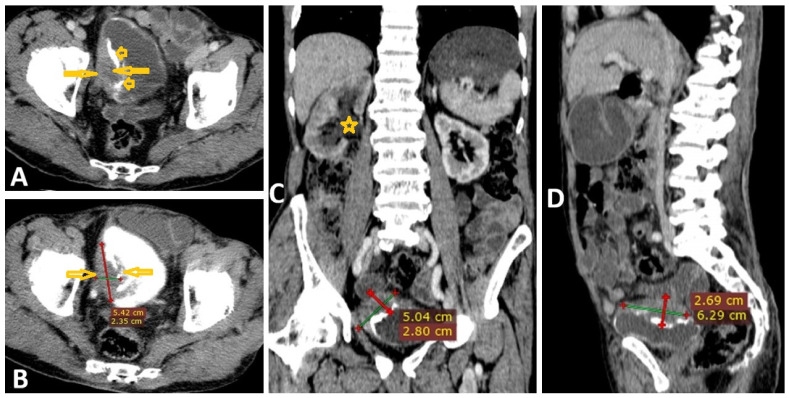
Selected computed tomography (CT) images of an 80-year-old male patient with a known case of chronic infection with *Schistosoma haematobium* who presented with frequent urination. CT images show a heterogeneously enhanced mass (arrow on axial image (A)) measuring about 63 × 54.2 × 28 mm infiltrating the right supero-lateral wall of the urinary bladder (UB) with foci of calcification (arrow heads on axial image (A)) in the wall of the UB. The mass appears as a filling defect in the UB with contrast axial images (arrows on (B)), which infiltrates the right vesico-ureteric junction, causing secondary right mild hydroureteronephrosis (star on coronal reconstruction image (C)). Sagittal reconstruction image (D) shows that the lesion infiltrates the perivesical fat with no infiltration into the muscle and bone of the pelvic wall, consistent with UB cancer.
